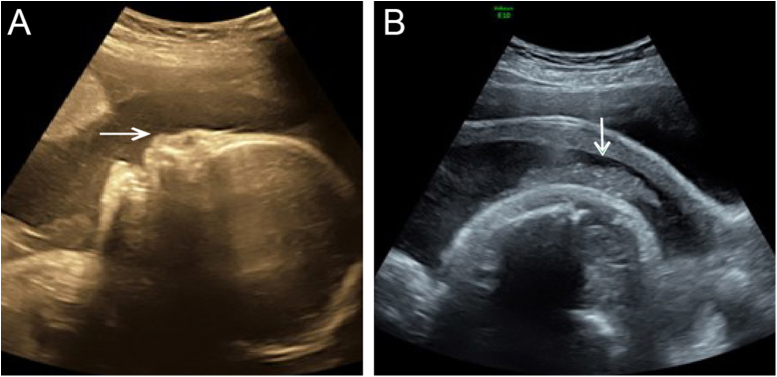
(A) 2-D ultrasound: The fetal face shows a flat curve and nasal hypoplasia (arrow) at the median sagittal section. (B) 2-D ultrasound: Abnormal skin flocculent echo (arrow)
